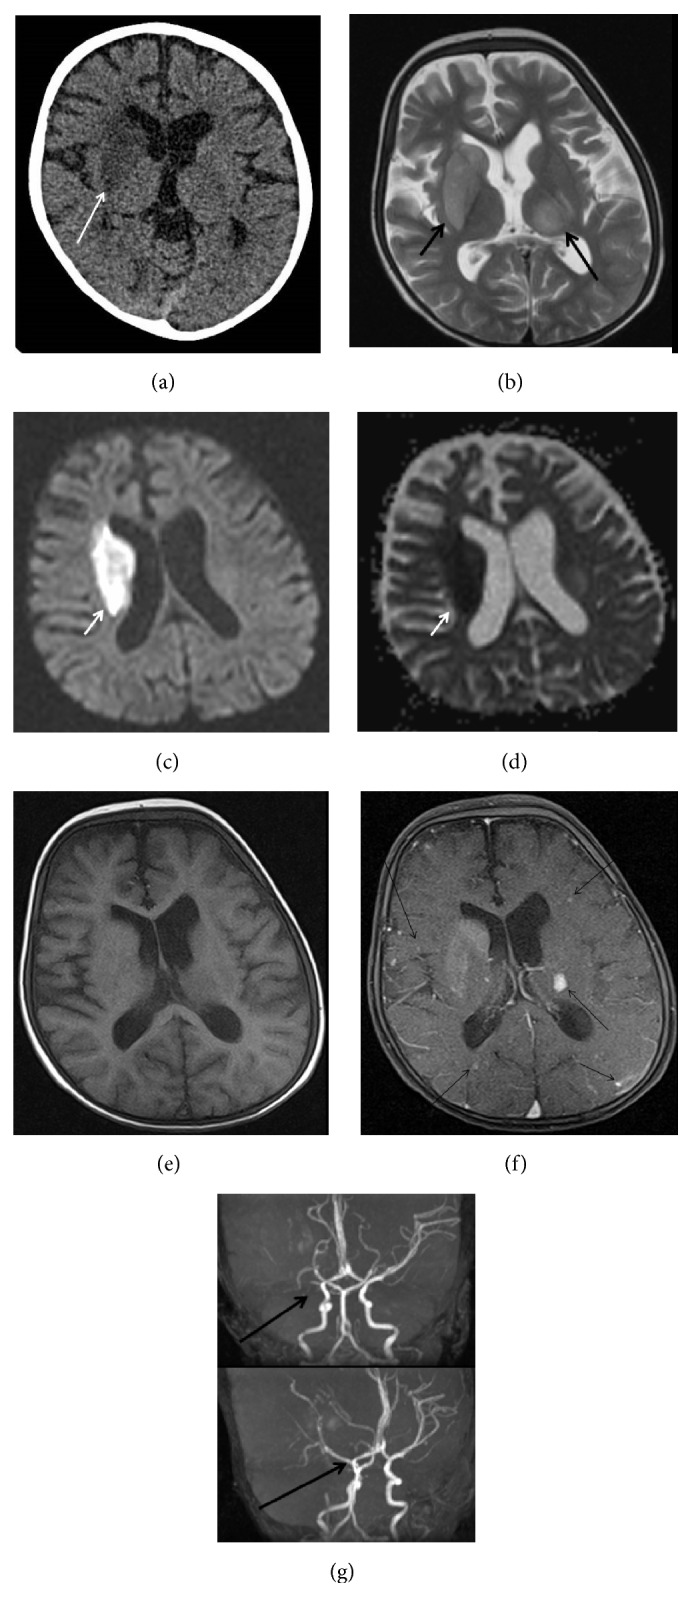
(a) Cranial CT: Hypodense area at the level of right basal ganglia compatible with acute ischemic infarction (arrow). (b) Cranial MR: Axial T2 image shows hyperintensity of right caudate nucleus, putamen, and globus pallidus. Contralateral thalamic hyperintensity is also seen (arrows). (c), (d) Diffusion restriction is seen at the level of right basal ganglia at diffusion b 1000 image and ADC map, respectively (arrow). (e), (f) Pre- and postcontrast T1 axial images show many enhancing intra-axial foci in both hemispheres after IV gadolinium administration (arrows). (g) Occlusion of right middle cerebral artery at the level of bifurcation on reconstructed MIP images of 3D TOF MR angiography.
